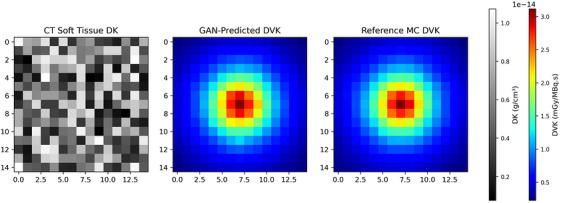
From left; CT soft tissue (15, 15, 15) density kernel, GAN‐predicted DVK and the Monte Carlo calculated reference DVK for the soft tissue.
